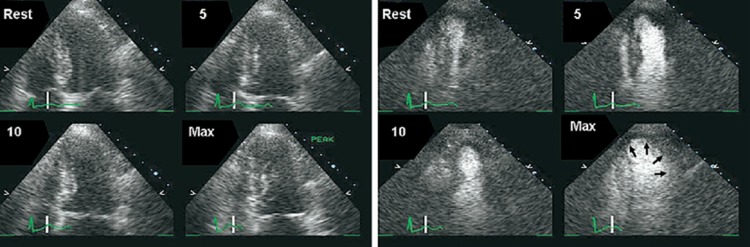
Intra-venous contrast agent use in echocardiography. Left, rest images have poorly defined endocardial border. Right, following administration of intra-venous contrast agent the apical wall motion defect is evident. Taken from Plana *et al*.^141^
